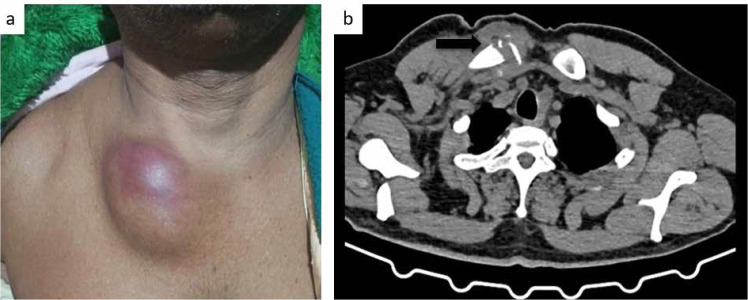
(A) Clinical picture showing erythematous swelling over right sternoclavicular joint region. (B) CT scan of the chest showing lytic lesions with adjacent few tiny bone fragments and oedematous soft tissue planes noted at the proximal end of the right clavicle.
